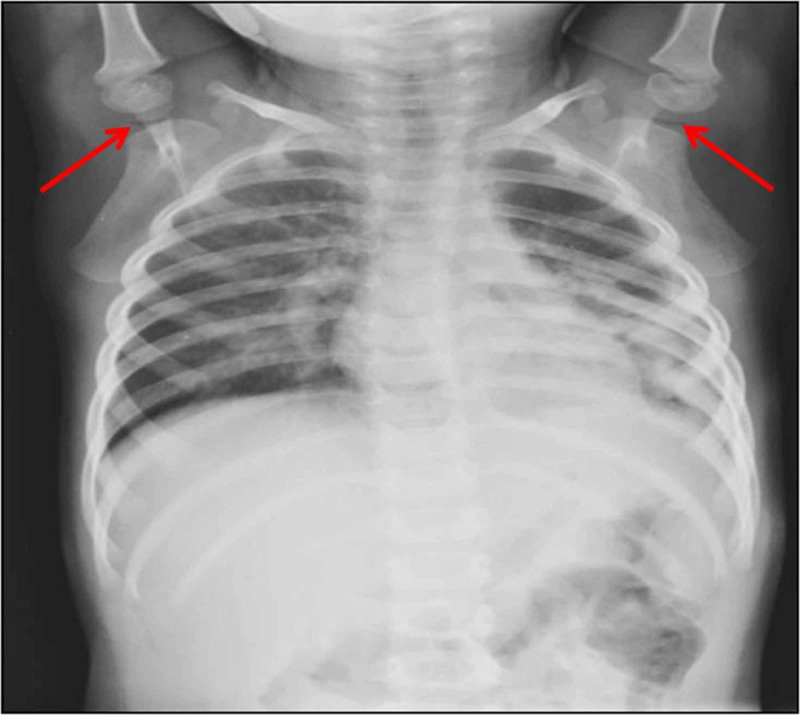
Chest radiograph of a three-year infant showing pneumoarthrosis in shoulder joints
